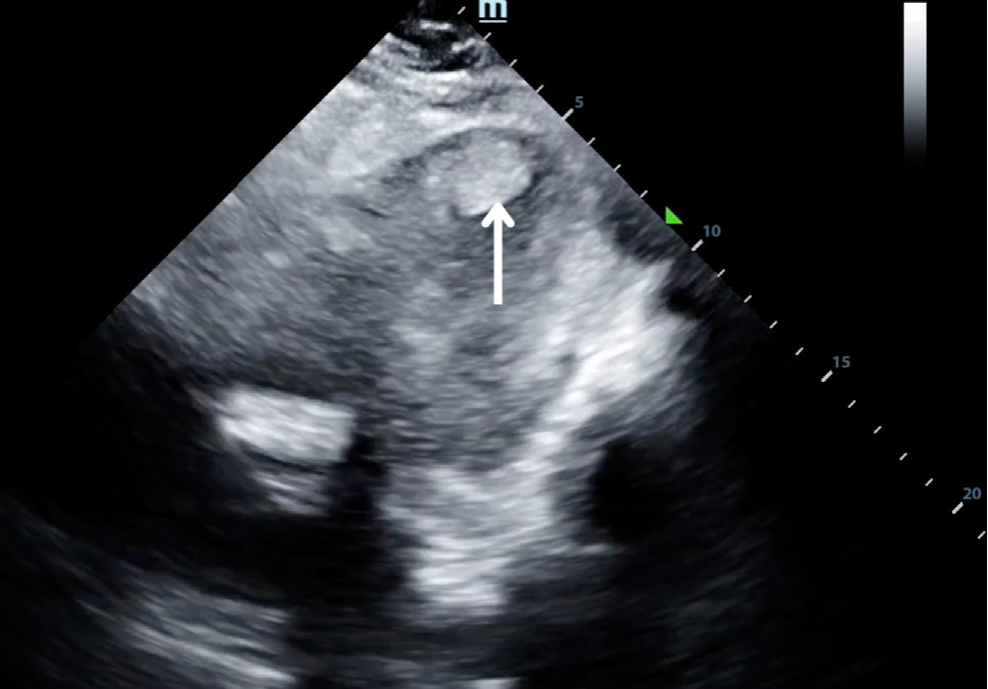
Sonographic apical cardiac view demonstrating a hyperechoic thrombus (arrow) in the apex of the left ventricle.
